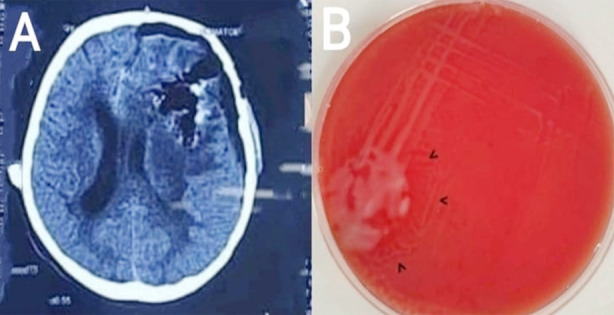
A) Non-contrast computerized tomography (CT) brain showing post-operative changes with no new pathology. B) Swarming motility of Proteus mirabilis on blood agar marked with black arrowheads.
